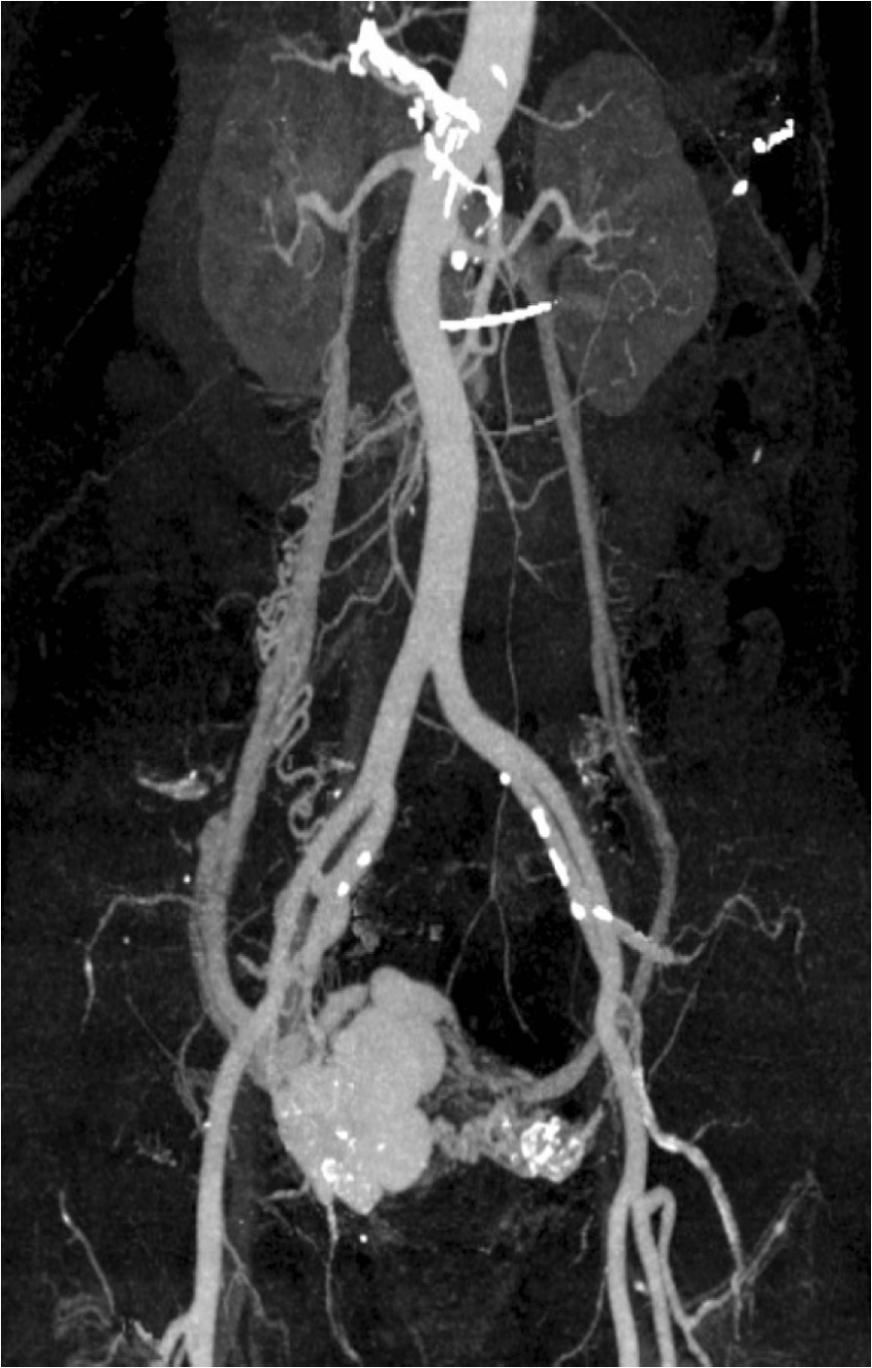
Computed tomography (CT) angiography suggests multiple expanded vessels around the uterus, including the right uterine artery, right internal iliac artery, right external iliac artery, and left uterine artery.
